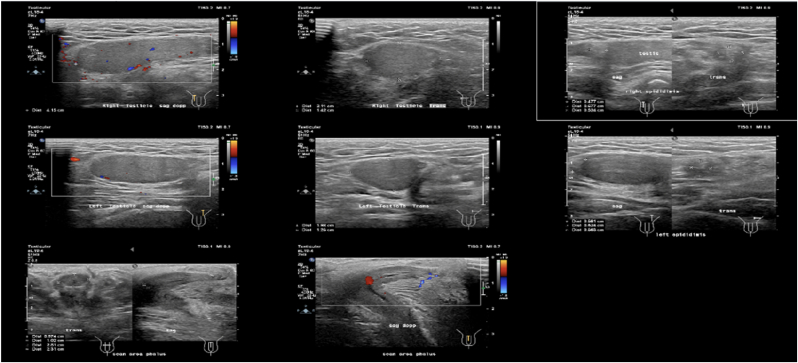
The result of Testicular Ultrasonography.
